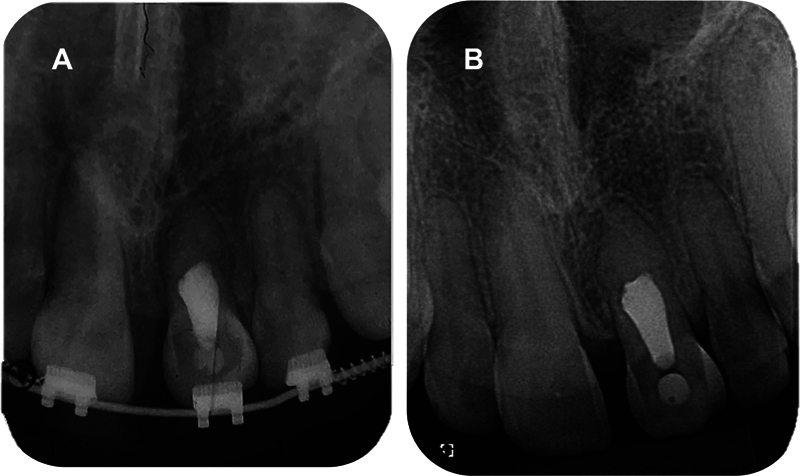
Follow-up periapical radiographies of the endodontic treatment. (
